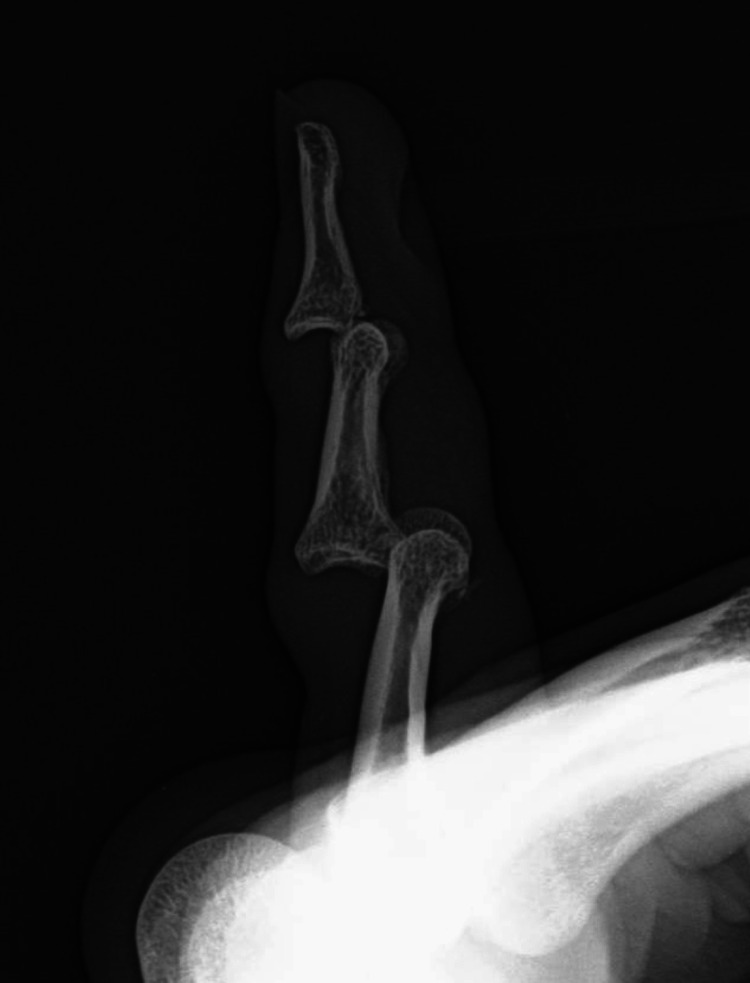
Lateral left little finger radiograph before reduction. Lateral radiograph of the patient’s left little finger showing double dislocation of both proximal and distal interphalangeal joints.
